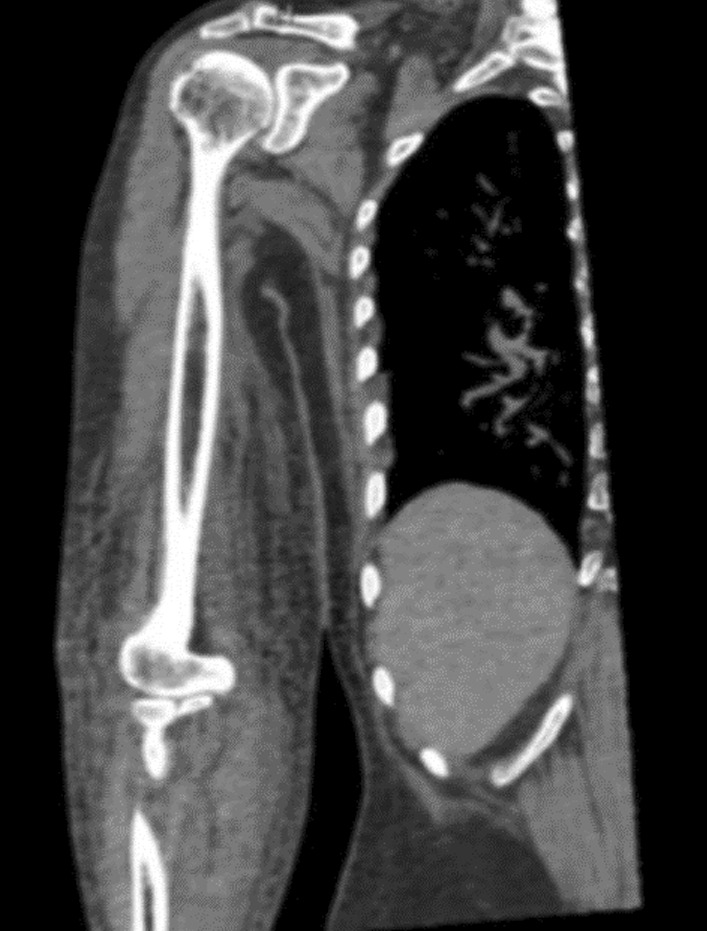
Computed tomographic examination of the right upper arm. A soft tissue opacity is seen in the right arm
